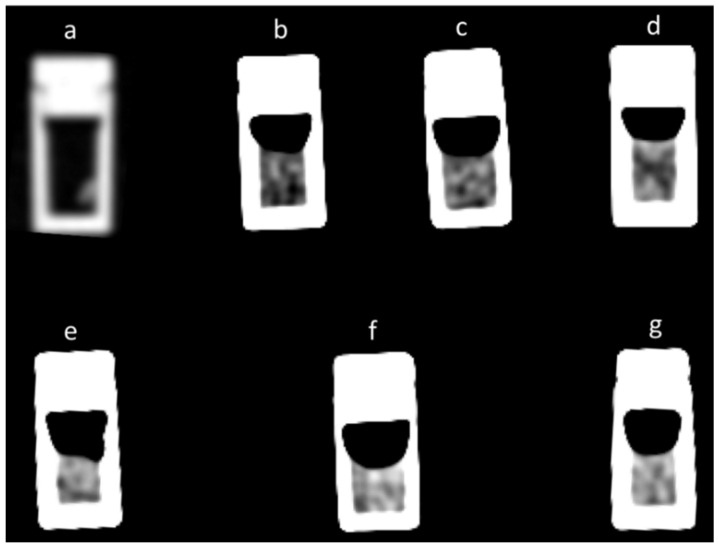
Computed tomography (CT) images in dual-energy (80 kV) mode of all seven different thrombus models taken five hours after initiation of their clotting: platelet thrombus (a), pure plasma thrombus (b), 20% red blood cell (RBC) thrombus (c), 40% RBC thrombus (d), 60% RBC thrombus (e), 80% RBC thrombus (f) and 100% RBC thrombus (g). In images (b–g), presented in the same Window Width (WW) and Window Center (WC), it is clearly seen how the image brightness increases with increasing HT. The platelet thrombus in image (a) is displayed with different WW and WC, which is optimal for its presentation.
